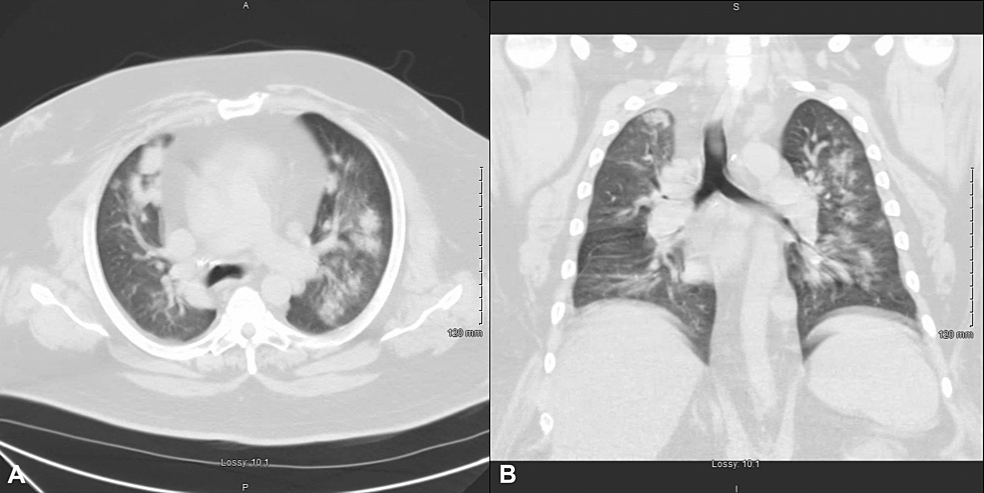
A) axial section of a chest computed tomography demonstrating bilateral patchy and nodular airspace infiltrates; B) coronal section of chest computed tomography demonstrating bilateral patchy airspace infiltrates
